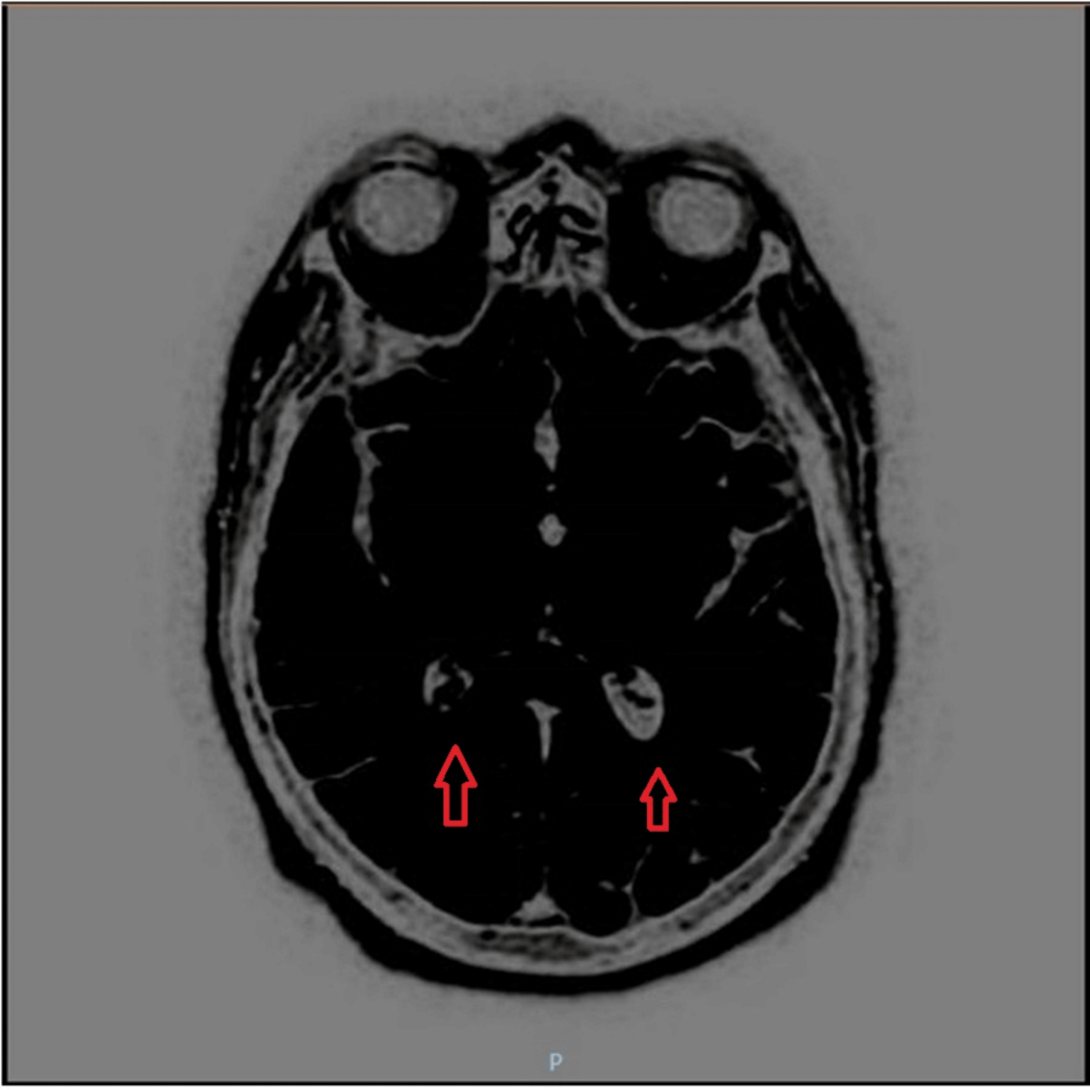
A contrast-enhanced MRI of the brain was conducted, revealing fluid levels with increased diffusion and FLAIR signal within the occipital horns of bilateral lateral ventricles, which was suggestive of pyogenic ventriculitis. FLAIR: fluid-attenuated inversion recovery; MRI: magnetic resonance imaging
